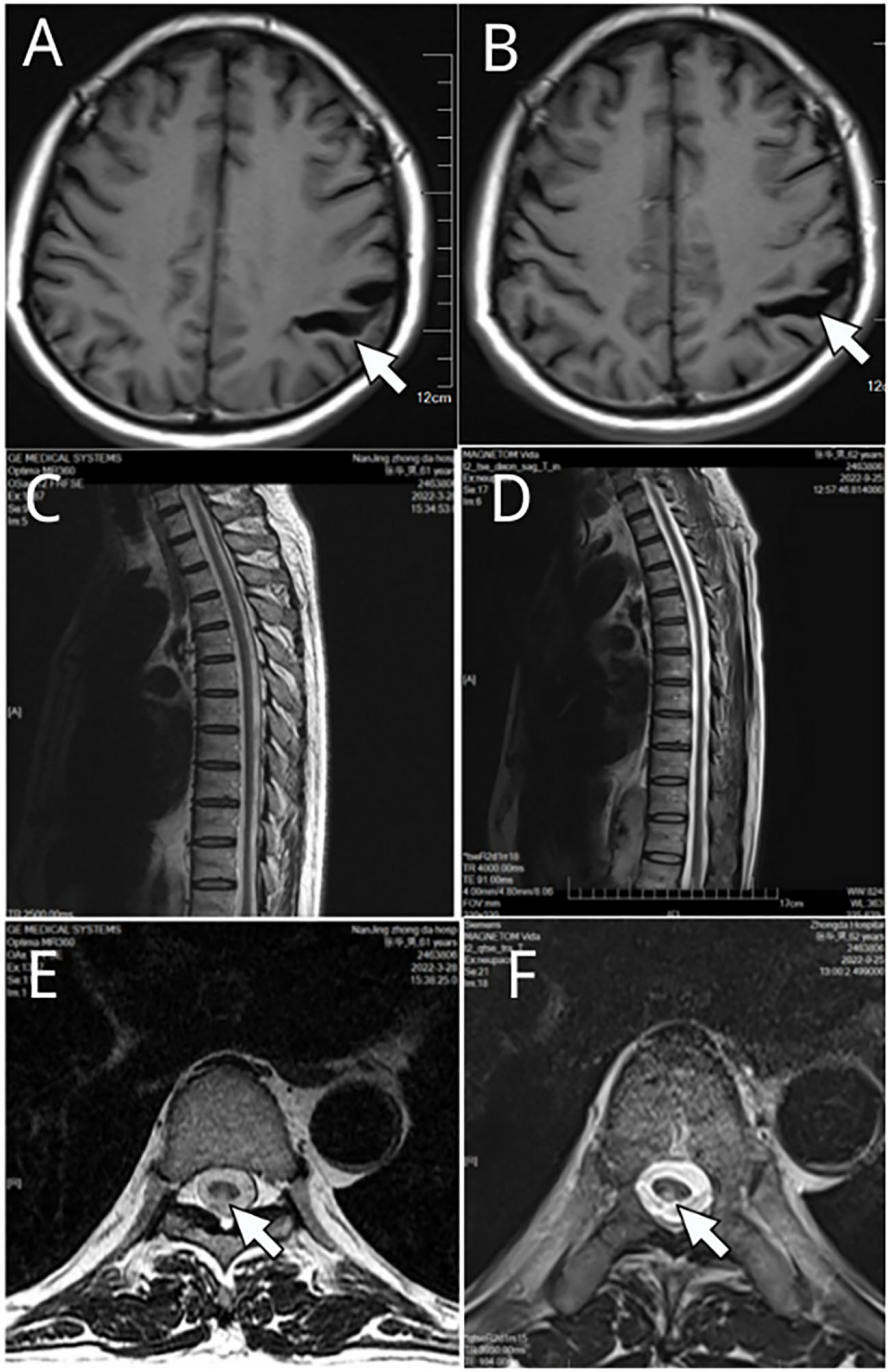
Nuclear magnetic resonance imaging (MRI) of the thoracic spine and cranial brain. (A, C, E) were results in Mar 2022, and (B, D, F) were examination results in Sep. 2022. A widening of the left parietal sulcus was observed in (A, B) (white arrow indicated). Slightly higher signal at the level of the posterior cord of the spinal cord (E, F) white arrow indicated) in the T3-6 segment (C, D) are shown.
